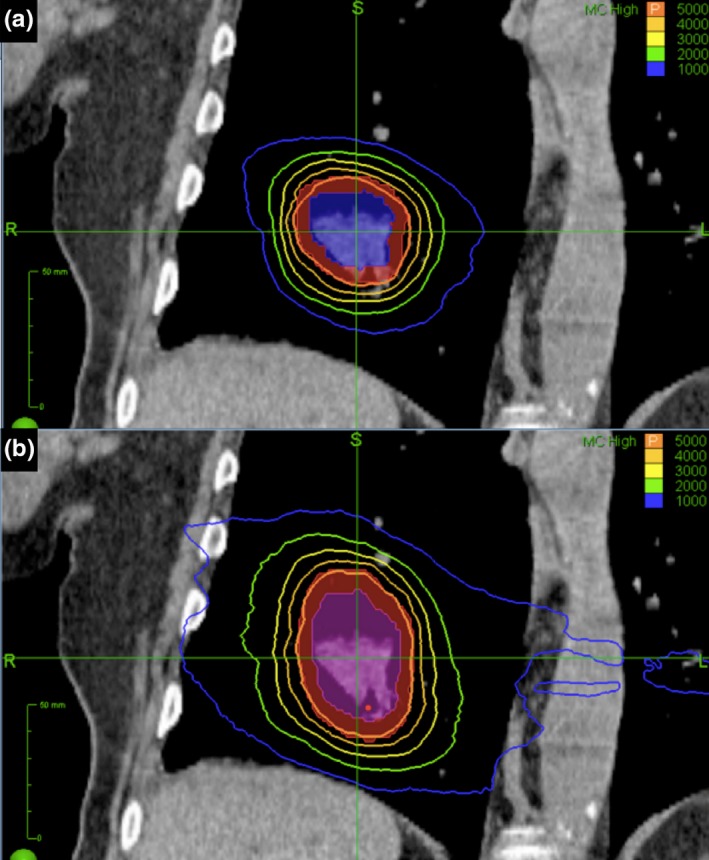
Example plan comparison with coronal slices of treatment planning CT for RTT plan (a) and ITV plan (b). GTV is shaded blue, ITV is shaded purple, and PTVs are shaded red. From RTT to ITV based‐plan, PTV volume increased 36.4 mL, PTV/lung volume ratio increased 0.69%, and NTCP increased 4.1%.
